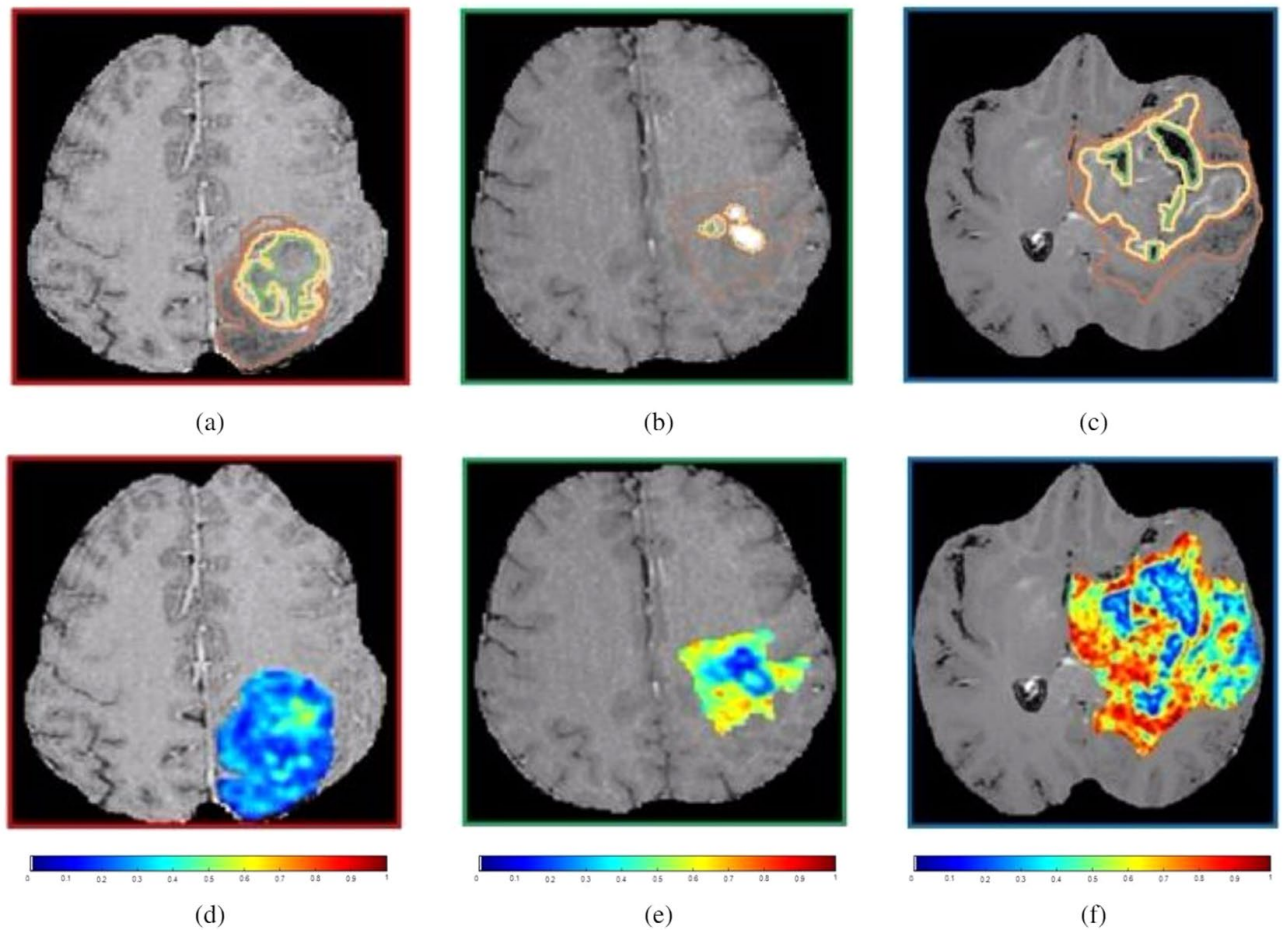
(a)–(c) show a 2D Gd-T1w MRI slice with expert-annotated necrosis (outlined in green), enhancing tumor (yellow) and edematous regions (brown) in 3 different GBM patients that exhibited low, medium, and high HES respectively. The corresponding Haralick feature map has been overlaid on the manually annotated tumor regions, for HES_*low*_ (d), HES_*medium*_ (e), and HES_*high*_ (f).
